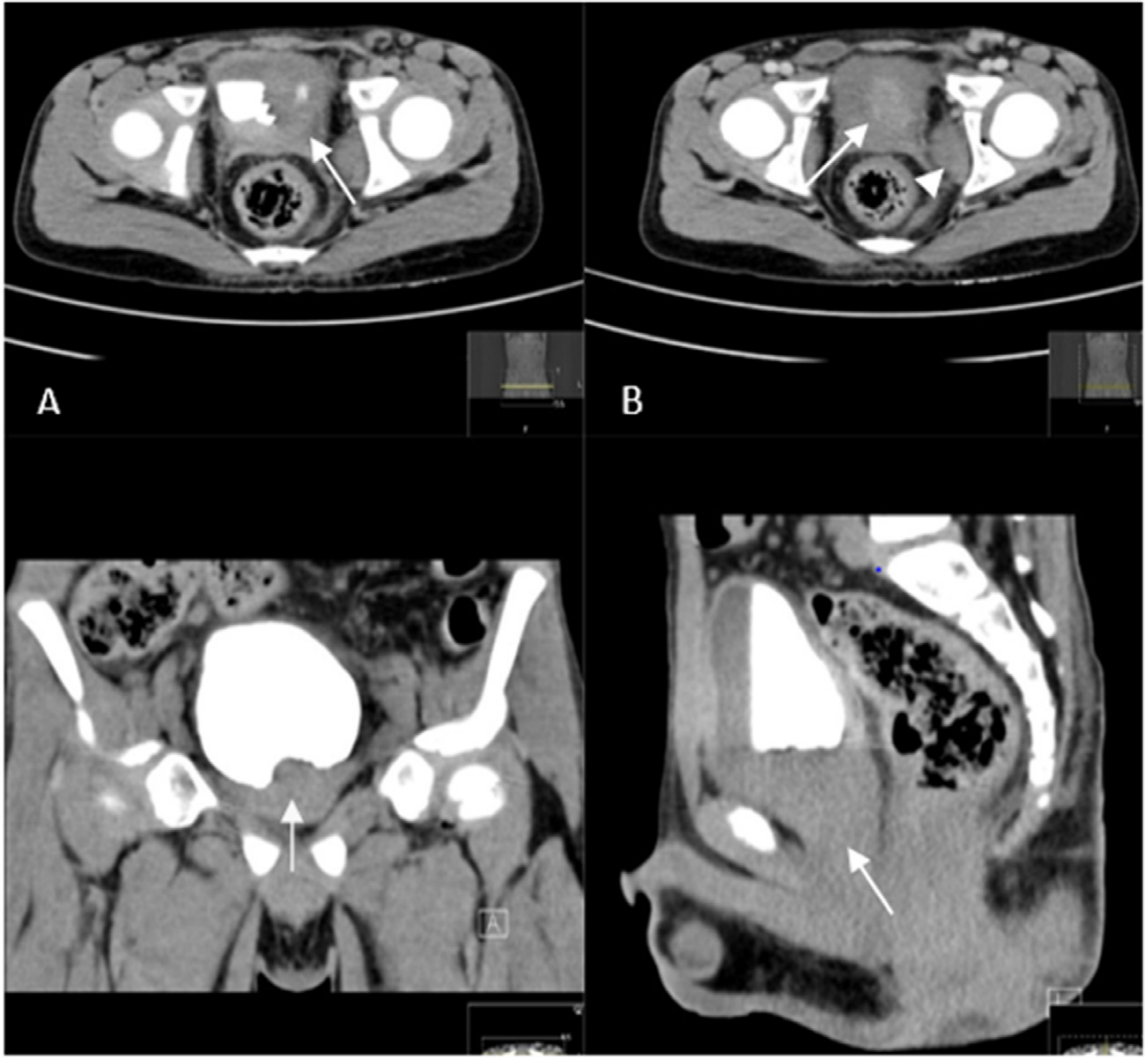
Axial section of delayed images CT scan showed (A) contrast filled bladder with partial filling defect at the level of bladder's trigone. (White arrow), and (B) mild hyperdense deep pelvic mass lesion inseparable from the prostate (white arrow) and enlarged left internal obturator muscle. (Arrow head). (C & D) Coronal and sagittal cut images showed deep pelvic mass lesion without distinct origin either from bladder or prostate, respectively. (White arrow).
